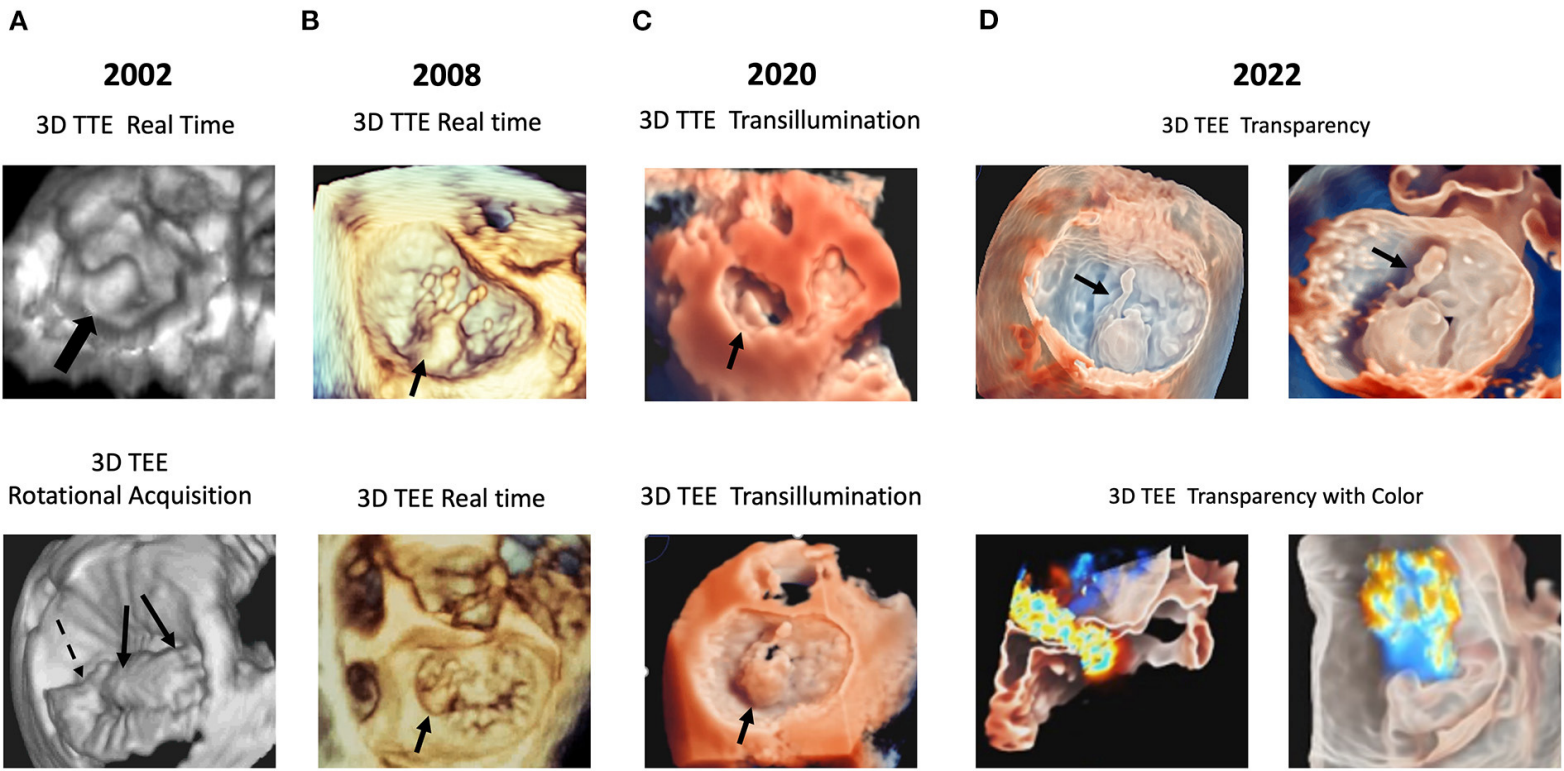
Examples of technical evolution of 3D echocardiography. In (A) examples are reported of P2 prolapse by RT 3D TTE (top panel); anterior and posterior leaflet prolapse (arrows) by rotational 3D TEE (bottom panel). In (B) examples are reported of P2 flail by RT 3D TTE (top panel), and P1 flail by 3D RT TEE (bottom panel). In (C) an example is reported of TI rendering: P2 prolapse by 3D TTE (top panel) and by 3D TEE (bottom panel). In (D) an example is reported of the “transparency” effect: P2 flail with a detailed visualization of the ruptured chorda by 3D TTE (top panel); mitral regurgitation color Doppler superimposed to the 3D TEE image (bottom panel). 3D, three-dimensional; RT, real-time. TEE, transesophageal echocardiography; TI, transillumination. TTE, transthoracic echocardiography.
